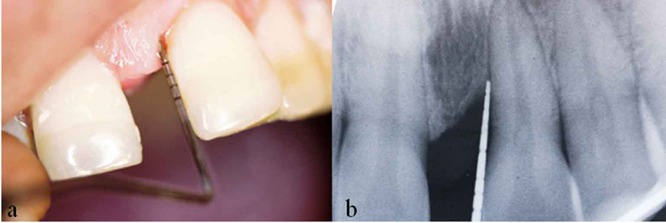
a: Pre-operative view of maxillary left central incisor shows 6mm pocket depth on mesial aspect b: Pre-operative radiographic view of maxillary left central incisor shows 5mm of intrabony defect
